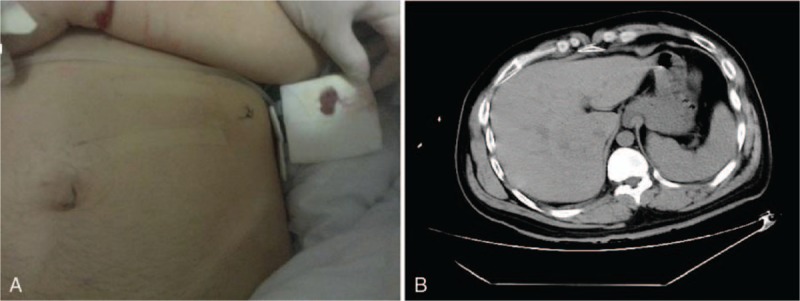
(A) Photograph of abdominal wound. (B) Computed tomography scan showing no evidence of abdominal wall perforation.
